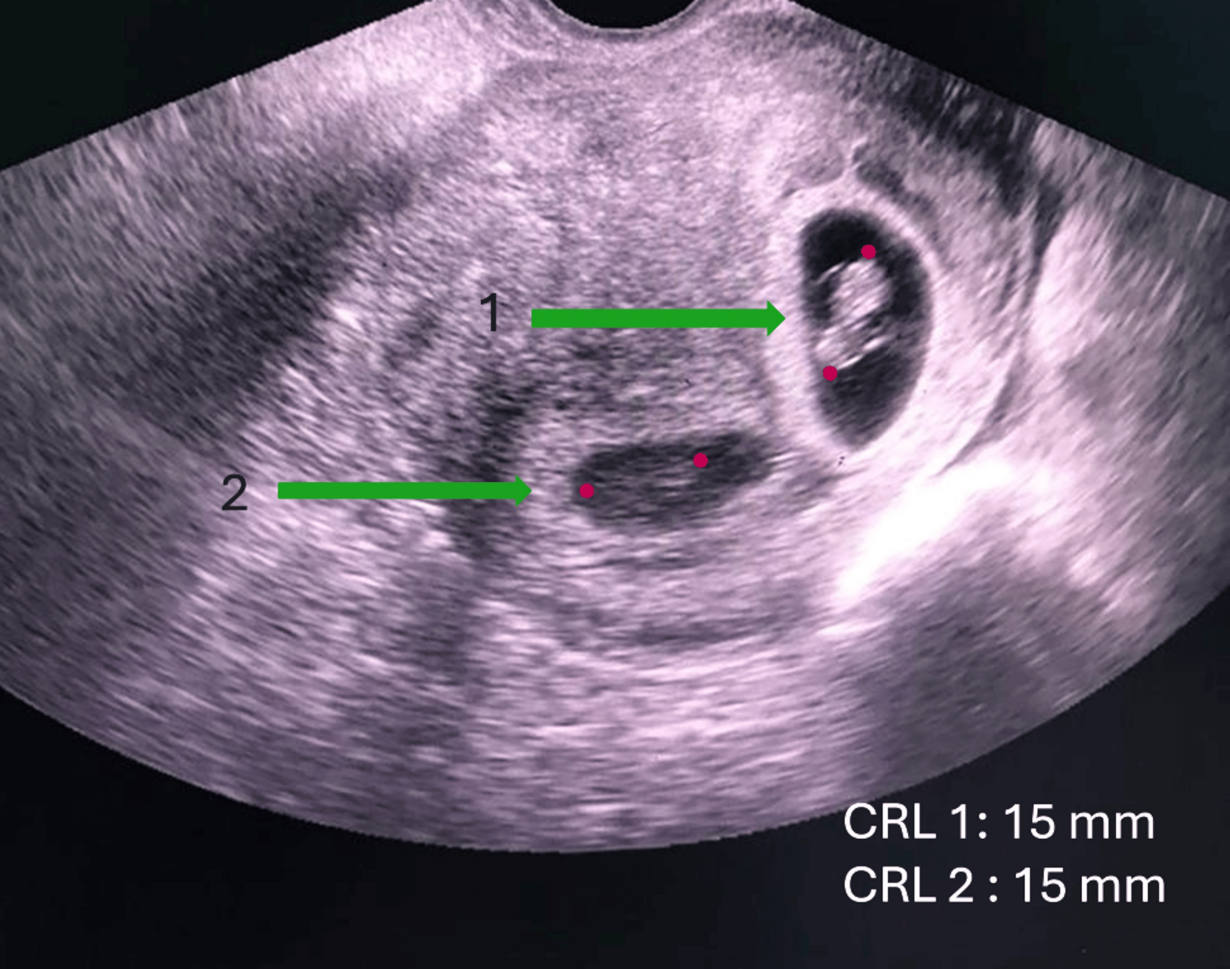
Transvaginal ultrasound shows the presence of two intrauterine gestational sacs with positive fetal echoes.
